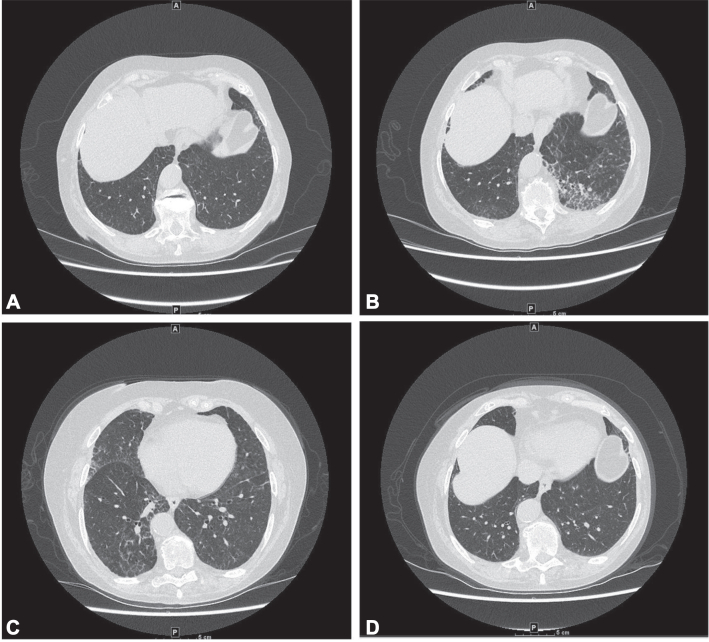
A, Chest high-resolution CT imaging shortly after the initial visit to ILD clinic showed mild reticulations in right lower lobe. B, Worsening interstitial changes with reticulation, mostly in the left lower lobe, despite removal of foam pillows. C, Improvements in the ground glass appearance and reticulations in left lower lobe, yet progressive changes in the right middle lobe 2 months after dupilumab cessation. D, Improvements in reticulations in the right middle lobe, right lower lobe, and left lower lobe 10 months after dupilumab cessation. *CT*, Computed tomography; *ILD*, interstitial lung disease.
